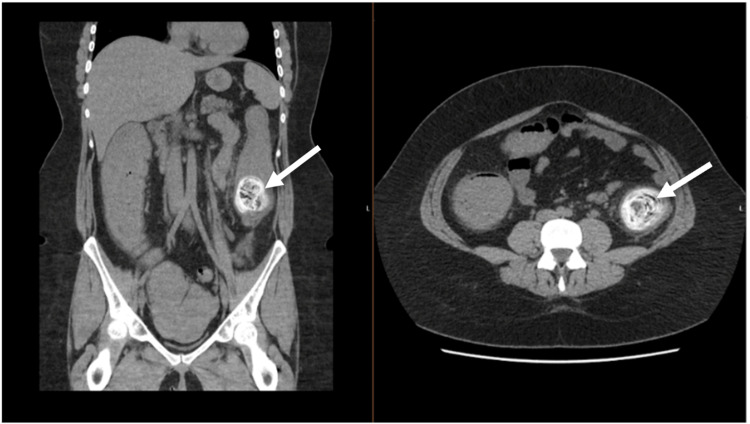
The patient's CT scan (case one) A coronal (left) and transverse (right) CT image of the patient's abdomen and pelvis shows a calcified faecolith in the proximal sigmoid colon.  An initial scan was performed on the patient's admission.
